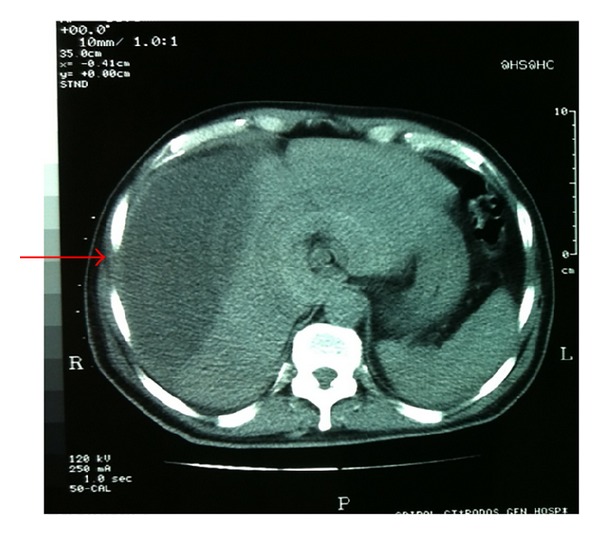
CT of the abdomen showing the subcapsular collection (arrow).
